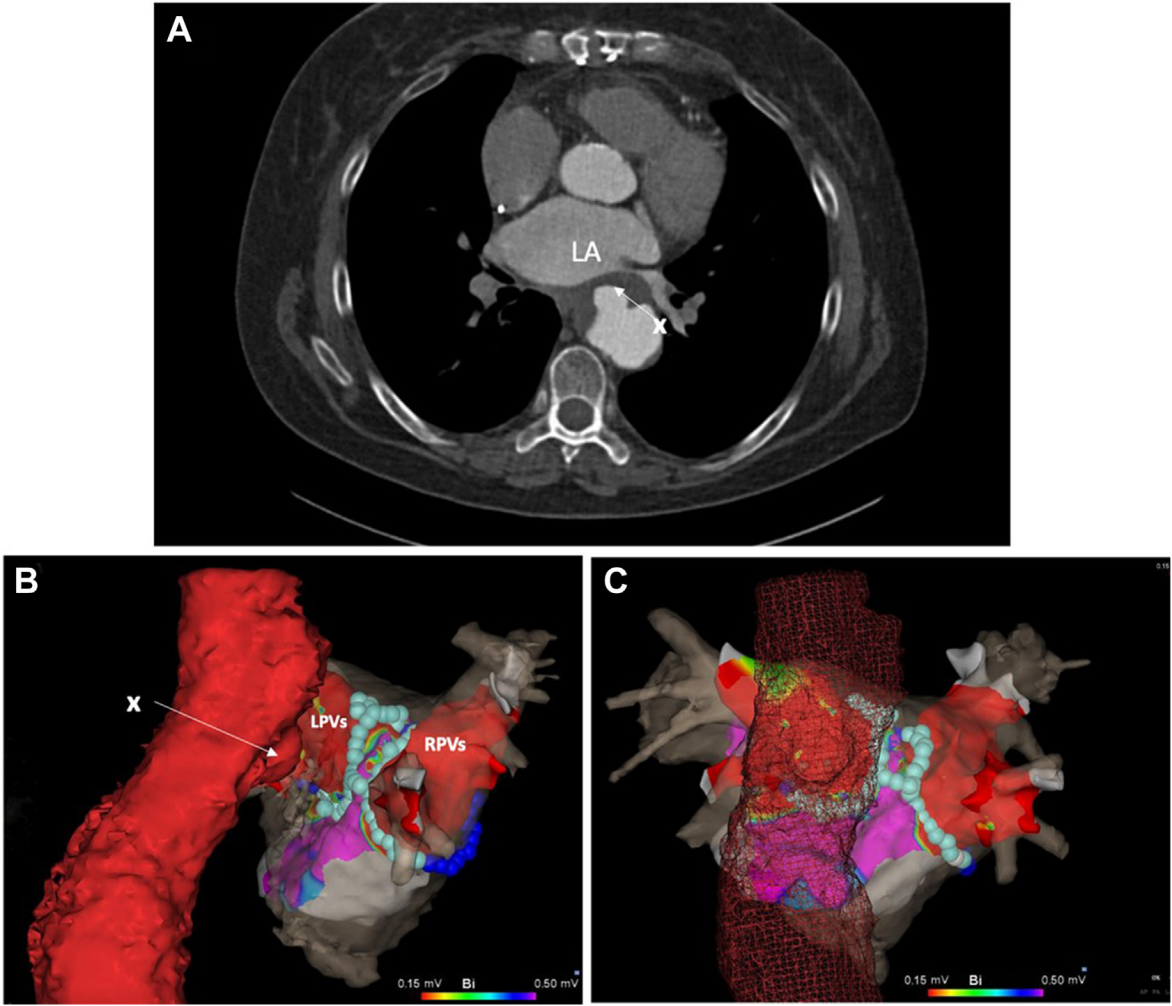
A: Cardiac computed tomography (CT) angiogram showing proximity between the saccular anterior aortic aneurysm and the left inferior pulmonary vein. B, C: Three-dimensional anatomical reconstruction integrating chest CT and 3-dimensional electroanatomical map (CARTO; Biosense Webster) demonstrating the proximity of the aneurysm to the applications of focal point-by-point pulsed field ablation (light blue balls) on the posterior wall of the left pulmonary veins (LPVs); right lateral (B) and posterior (C) views. LA = left atrium; RPVs = right pulmonary veins.
